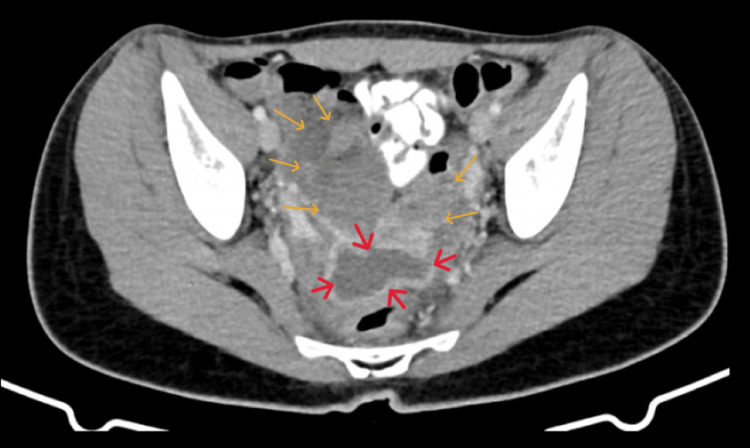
Computed tomography imaging of the periappendiceal abscess misdiagnosed as pyosalpinx An organized, thick-walled fluid collection with a spindle-shaped morphology (red arrows) is clearly visualized, along with increased opacification, diffuse fat exudation in the pelvis, and edematous imaging of the rectosigmoid (yellow arrows).
